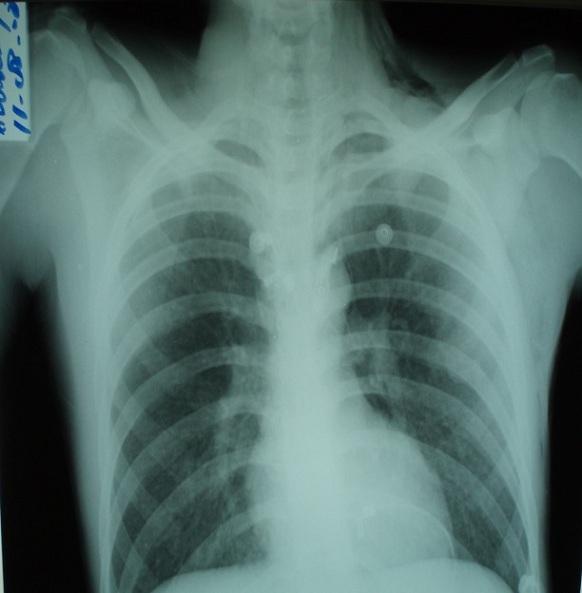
radiographie thoracique montrant le pneumomediastin: une fine hyperclarté linéaire silhouettant le bouton aortique, l’aorte descendante et le cœur
